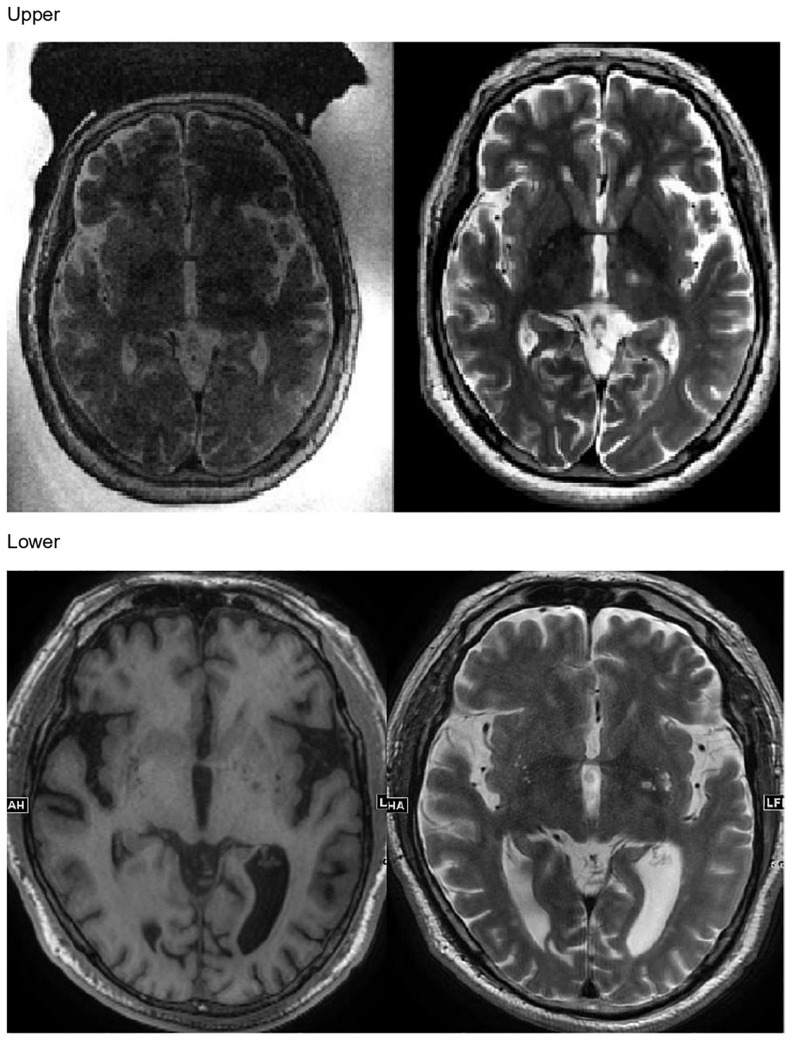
Upper: Magnetic resonance (MR) images taken immediately after MR-guided focused ultrasound autofocusing echo imaging thalamotomy in a patient with essential tremor. Lower: MR images taken immediately after MR-guided focused ultrasound autofocusing echo imaging pallidotomy in a patient with Parkinson’s disease.
